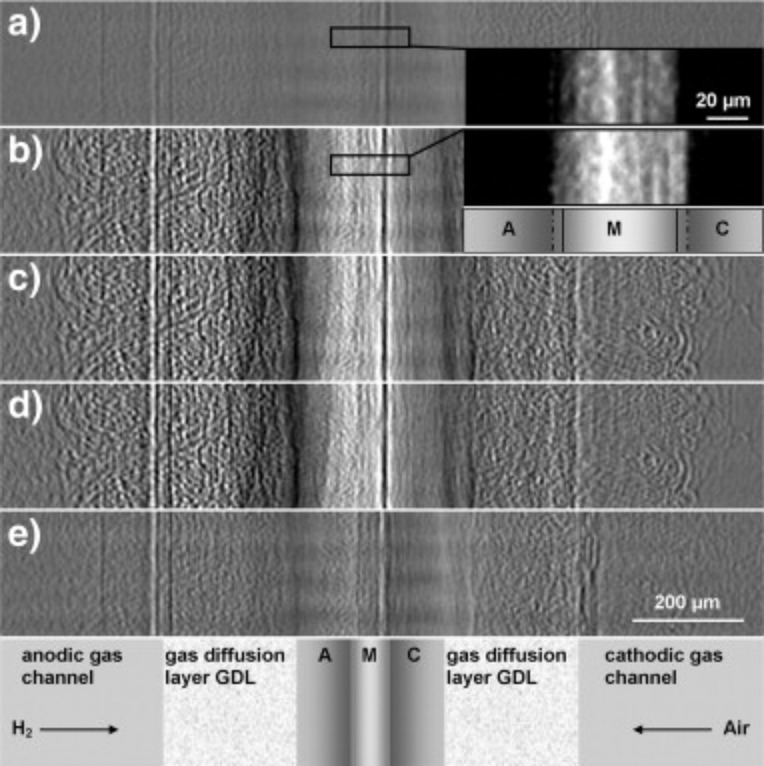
Normalized radiographs of the cross section of the MEA at different current densities *j*: a) 0 mA·cm^−2^ (OCV before), b) 140 mA·cm^−2^, c) 300 mA·cm^−2^, d) 550 mA·cm^−2^ and e) 0 mA·cm^−2^ (OCV after). Inset: Non-normalized enlarged radiographs of the membrane and parts of the catalyst layers at OCV and *j* = 140 mA·cm^−2^ (A, anode; M, membrane; C, cathode). Reprinted with permission from [65]. Copyright 2010 Elsevier.
